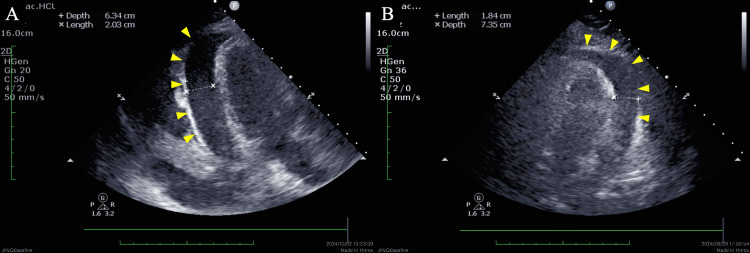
Echocardiography in emergency departments Echocardiography (A, B) shows massive pericardial effusion around the left ventricle (arrowheads).
